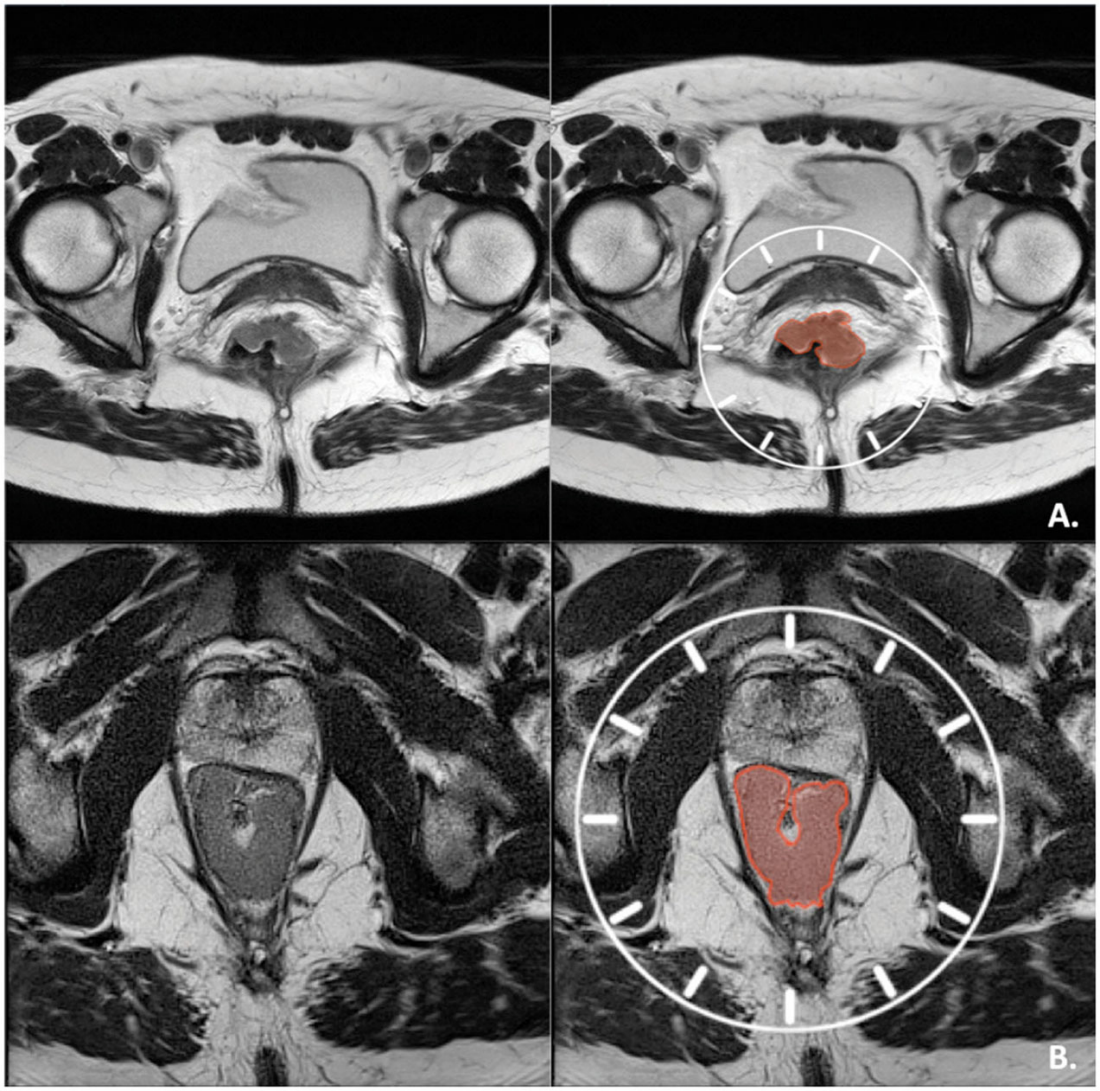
Tumor orientation. (A) Axial T2-weighted view of an anterior mrT3 tumor with center and invasive component between 11 and 1 o'clock. (B) Axial T2-weighted view of a non-anterior, early mrT3 tumor with center and invasive component between 5 and 7 o'clock.
